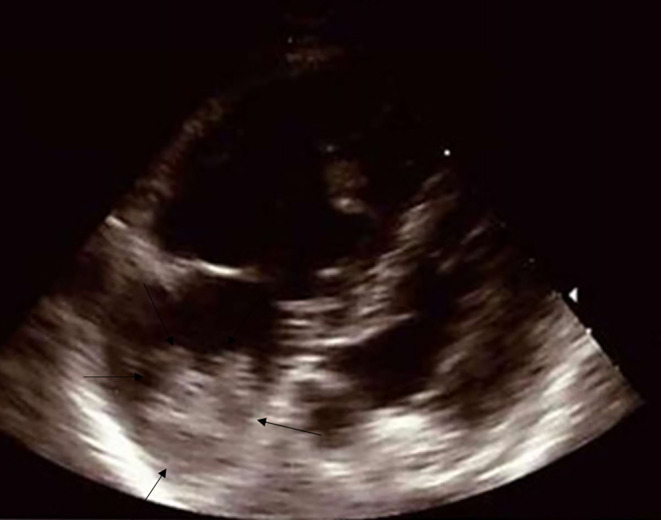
Transthoracic echography showing a floating structure in the right atrium
